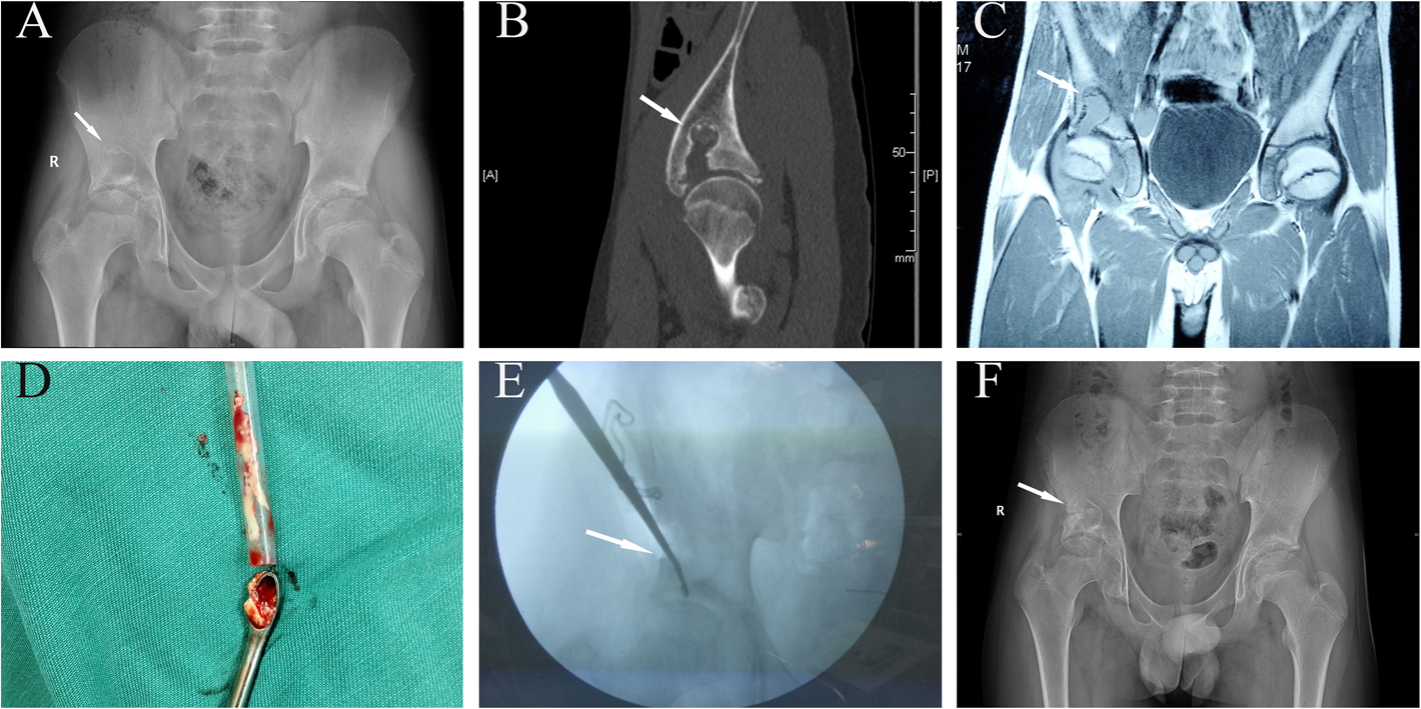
a-f A 17.3-year-old male patient (case N). The X-ray and CT results show right iliac bone destruction, with partial absence of the acetabulum, a clear boundary, and a sclerotic margin (a, b). MRI shows slightly long T1 signals in the right acetabular roof (c). During the operation, the lesion was found to consist of caseous tissue (d), and the X-ray results show that the lesion was cleared (e). The X-ray (f) results show partial bone growth after 6 months (white arrows indicate the site of the lesion)
